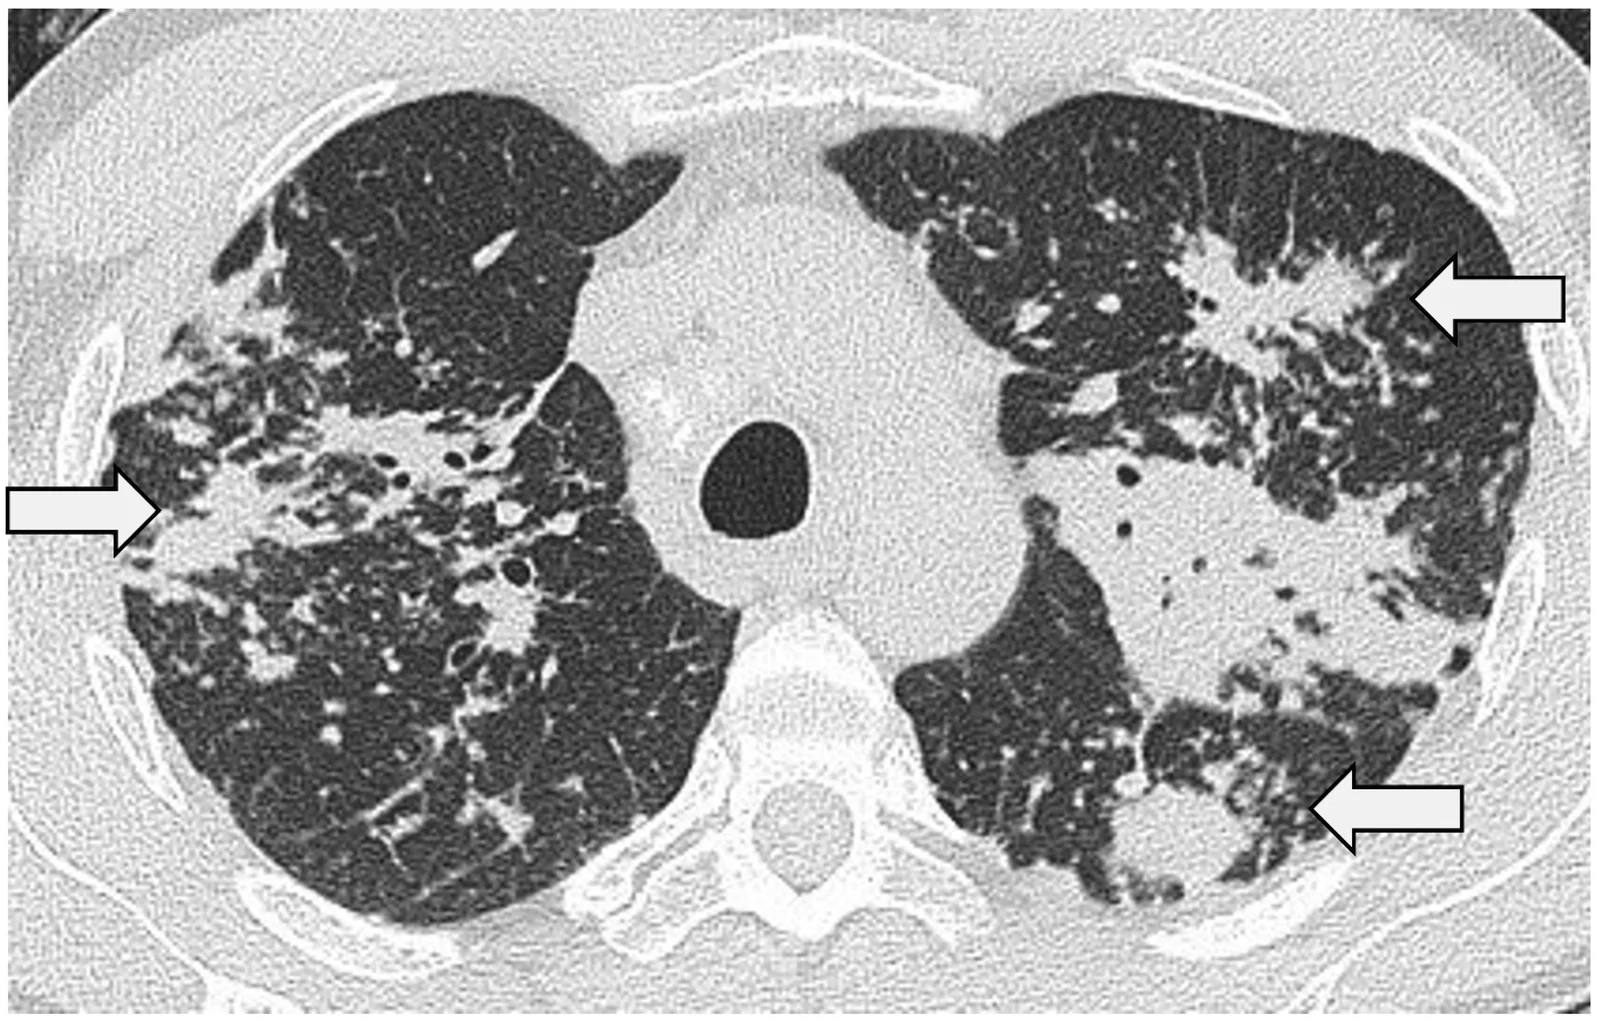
Radiographic findings of pulmonary sarcoidosis. Chest CT demonstrating bilateral upper lobe pulmonary nodules with surrounding clusters of micronodules (white arrows), findings consistent with sarcoidosis. Credit: Image adapted from Bailey et al. [5], J Clin Med. Used under the CC BY 4.0. CT, computed tomography
